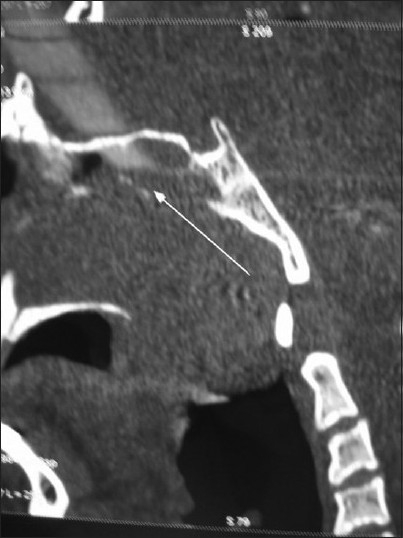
CT scan sagittal reconstruction demonstrating a soft tissue mass (chordoma) extending to the pre clival space and the extent of destruction at the upper part of the clivus and the sphenoid sinus
